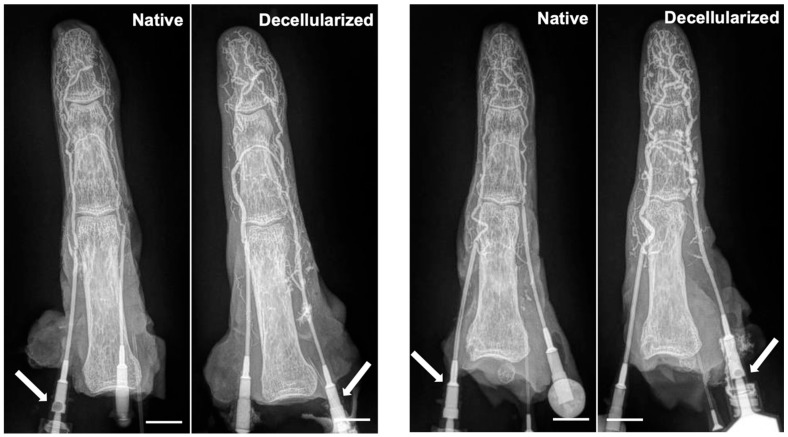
Digital X-ray with contrast images of native and decellularized digits. Two representative digits (left and right) are shown. Scale bars represent 1 cm. Arrow depicts artery through which contrast fluid was injected.
